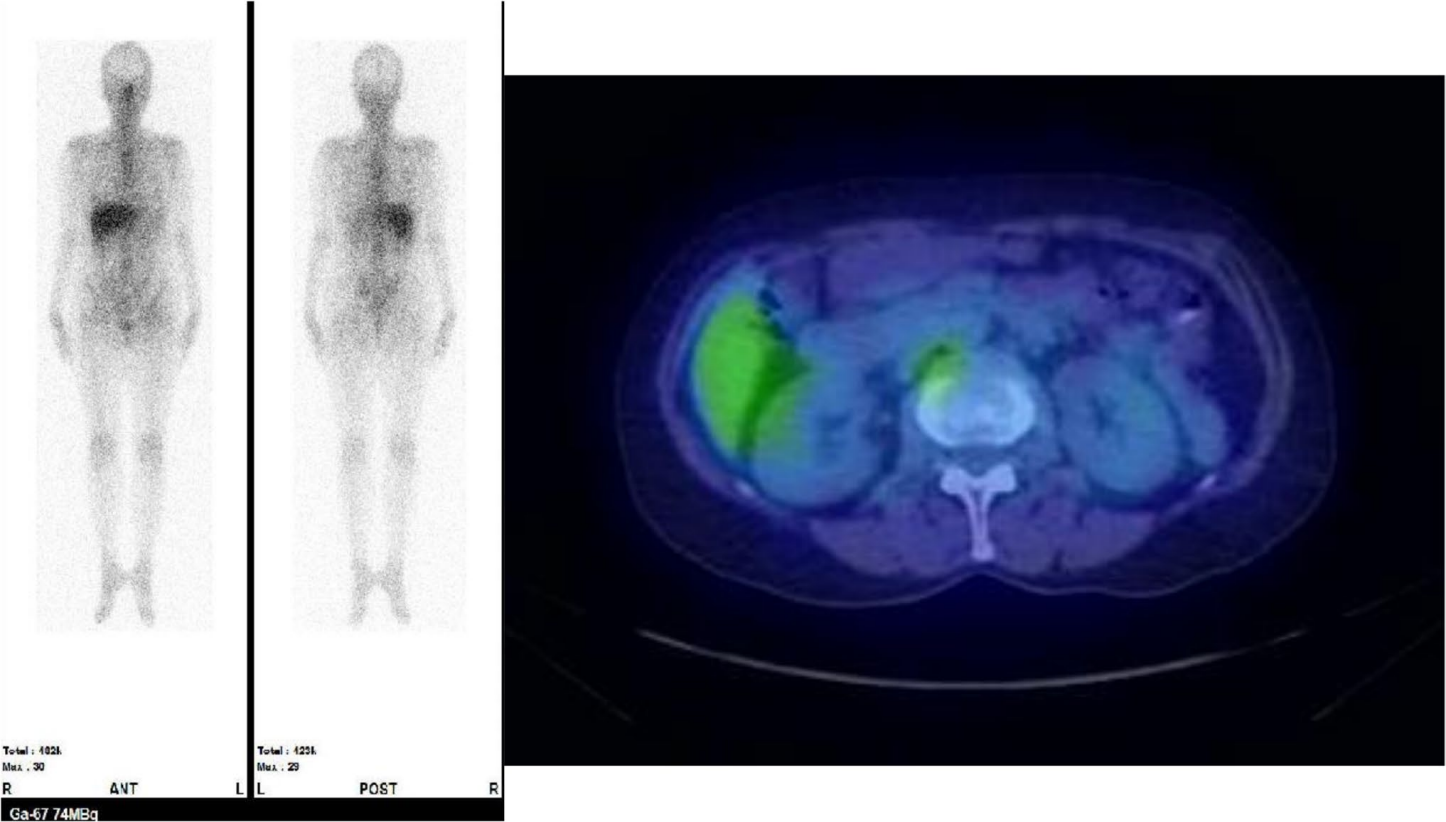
Gallium-67 scintigraphy images (planar coronal image and single-photon emission computed tomography [SPECT] horizontal image). Both images were negative in Case 1
